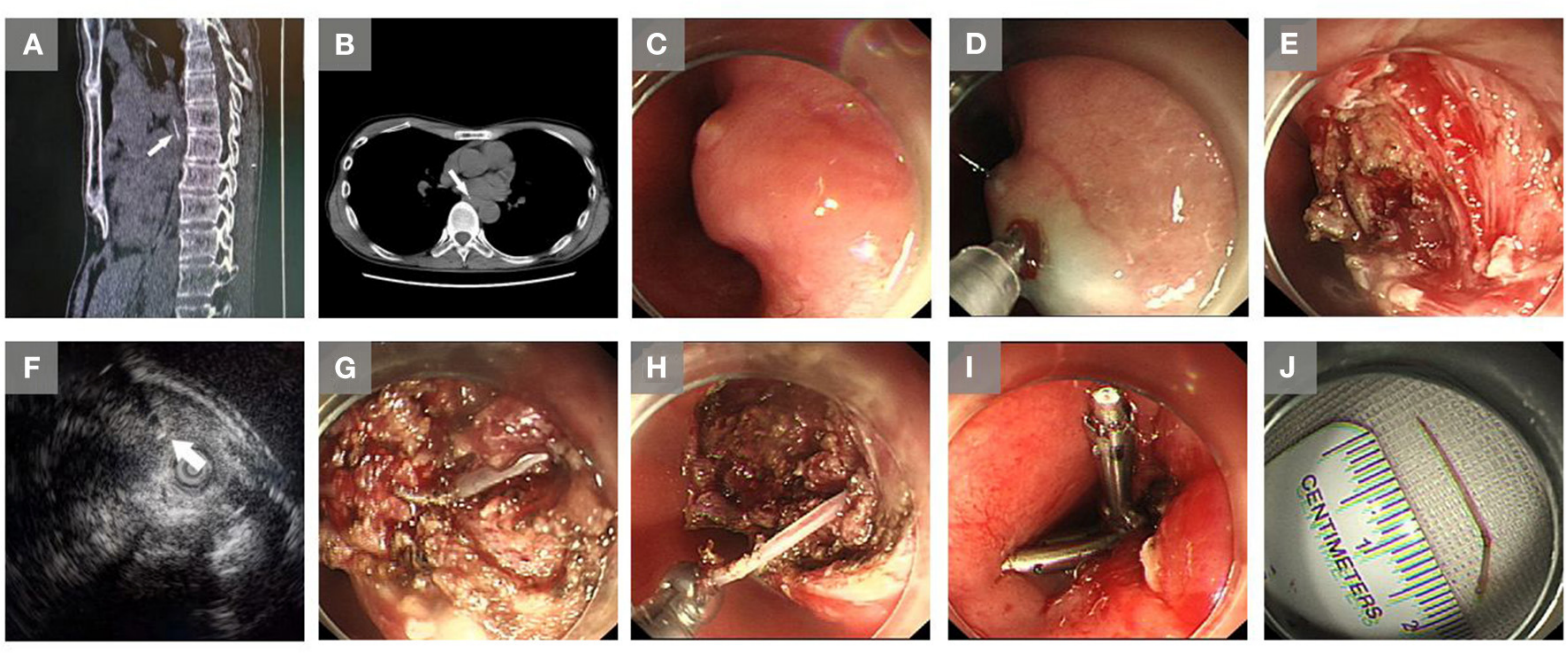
Images of the embedded foreign body in the esophagus, and extraction *via* endoscopic submucosal dissection in Case 1. (A) A buried foreign body (arrow) in the esophagus was revealed by a computer tomography (CT) scan at the local hospital. (B) A buried foreign body (arrow) in the esophagus was revealed by the CT scan at our hospital. (C) A mucosal protrusion with a small ulcer was observed with endoscopy. (D) A submucosal injection was performed around the mucosal protrusion. (E) Dissection of the mucosa and submucosa. (F) Endoscopic ultrasound imaging showed a fish bone-like linear hyperechoic mass. (G) Entirely exposed foreign body (a fish bone). (H) Removal of the foreign body (a fish bone) by forceps. (I) The wound was closed by using two metal clips. (J) The removed fish bone.
